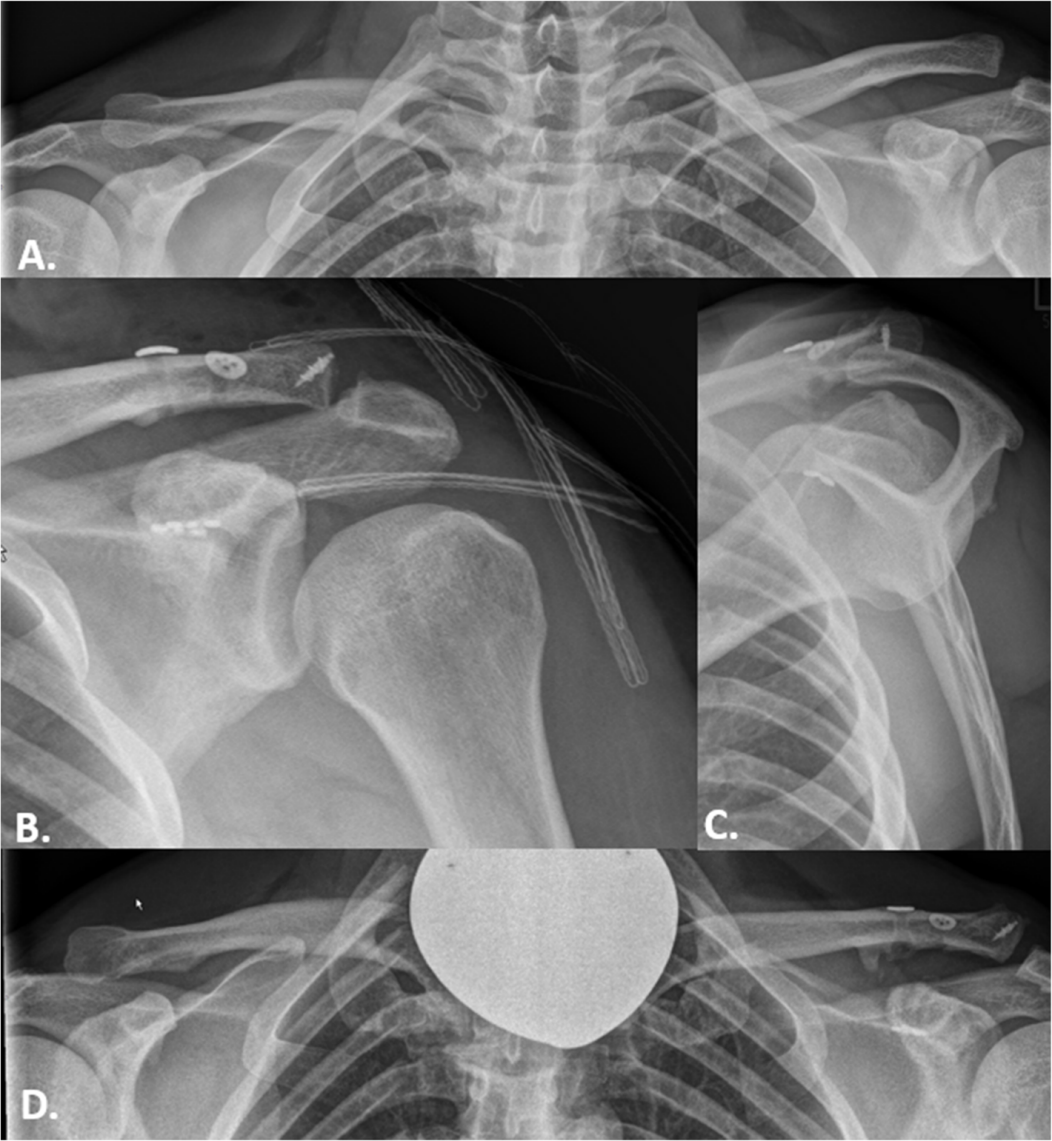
Case presentation. Left: A 42-year-old patient with an acromioclavicular joint injury due to a contact sport. A. A panoramic radiograph shows a Rockwood type IIIb injury. B and C. Radiographs obtained in the immediate postoperative period show the correct reduction of the ACJ (B. anterior-posterior and C. Alexander view). D. A radiograph obtained 67 months postoperatively shows good reduction with subtle ossification in the ligament area. The implants are without secondary dislocation (panorama view)
